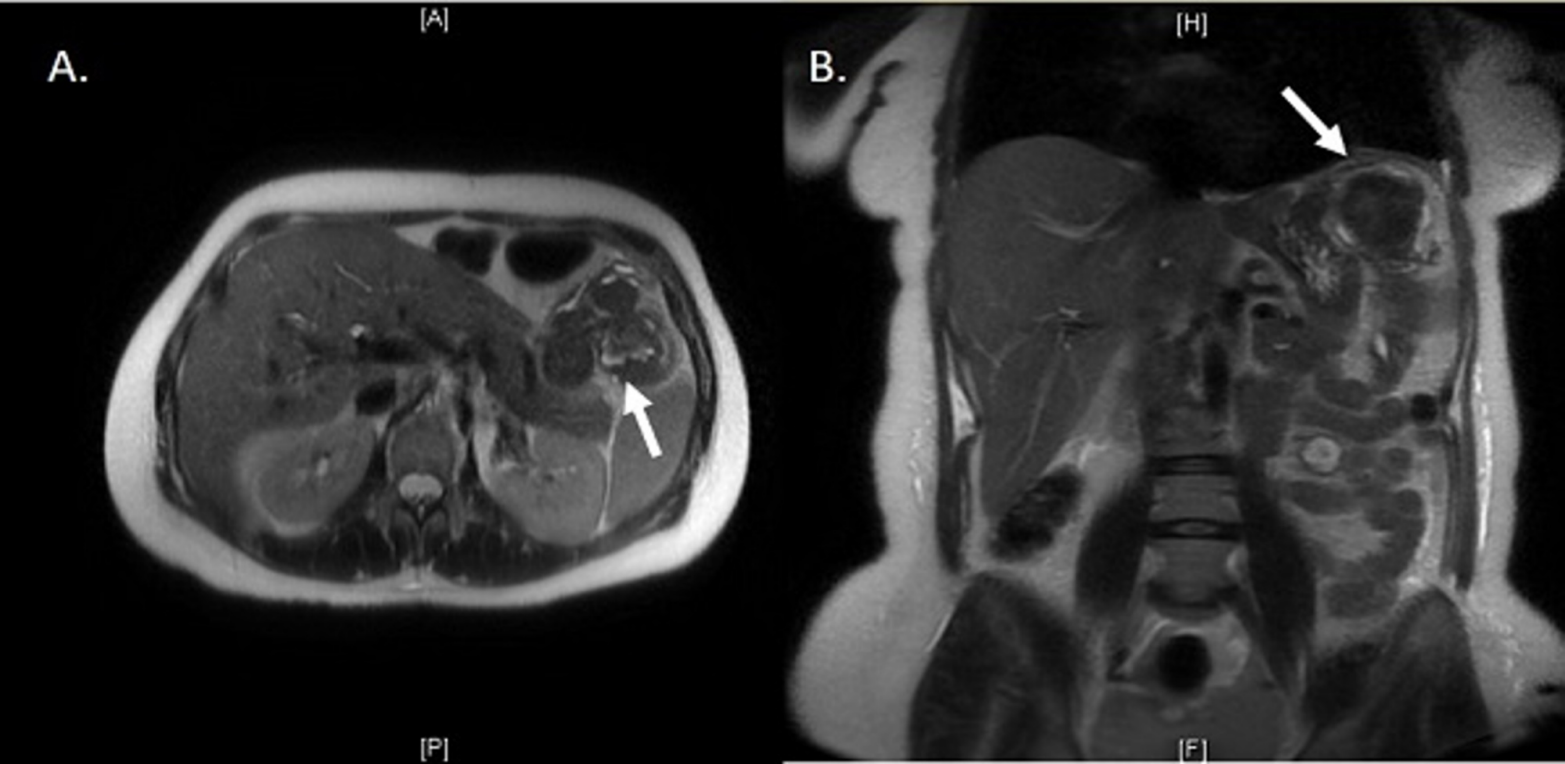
T2 weighted MRI of the abdomen demonstrated a hypoenhancing mass measuring 4 x 7.2 x 8 cm in maximum anteroposterior, transverse, and craniocaudal dimensions (arrow). (A) transverse view, (B) coronal view.
